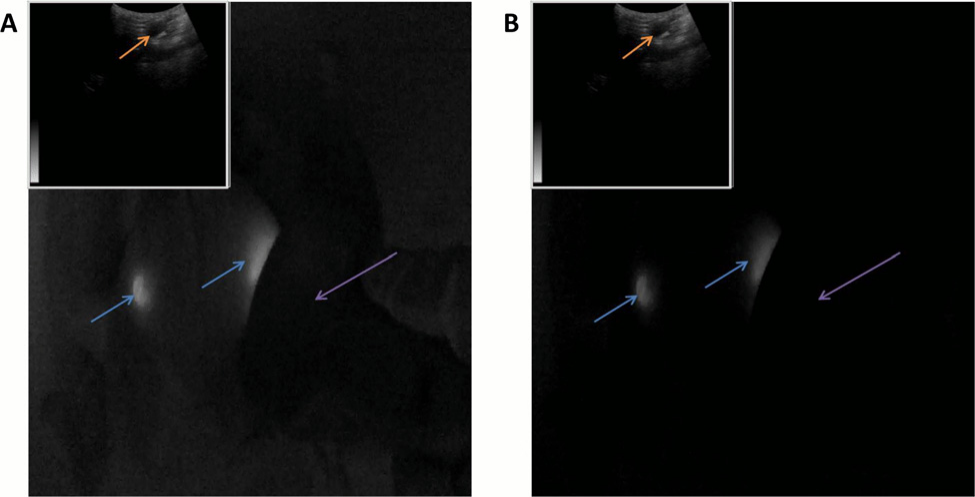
Goggle aided stereoscopic imaging with ultrasound. 2 fluorescent targets (blue arrows) implanted into the chicken breast at depths of approximately 3 mm. Ultrasound imagery is displayed in picture-in-picture mode at the upper left of the goggle imaging frame. The ultrasound transducer (purple arrow) was capable of detecting the implanted fluorescent tube as a dark region (orange arrow), similar to a large vessel or fluid filled sac. Imaging was conducted with (A) and without (B) unfiltered NIR illumination.
